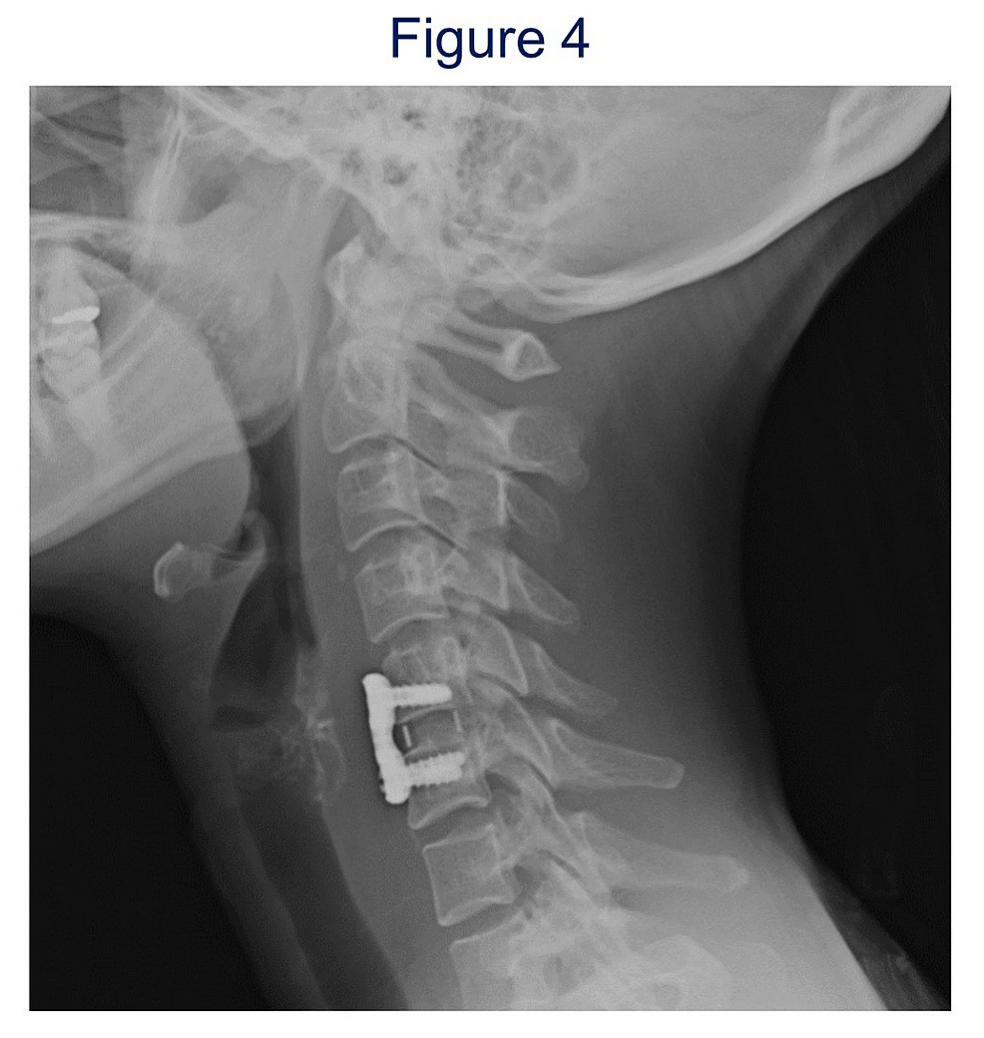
Postoperative lateral radiograph of case 2 (seven years after the surgery). C5-6 anterior fixation with cage and anterior plate was performed.
